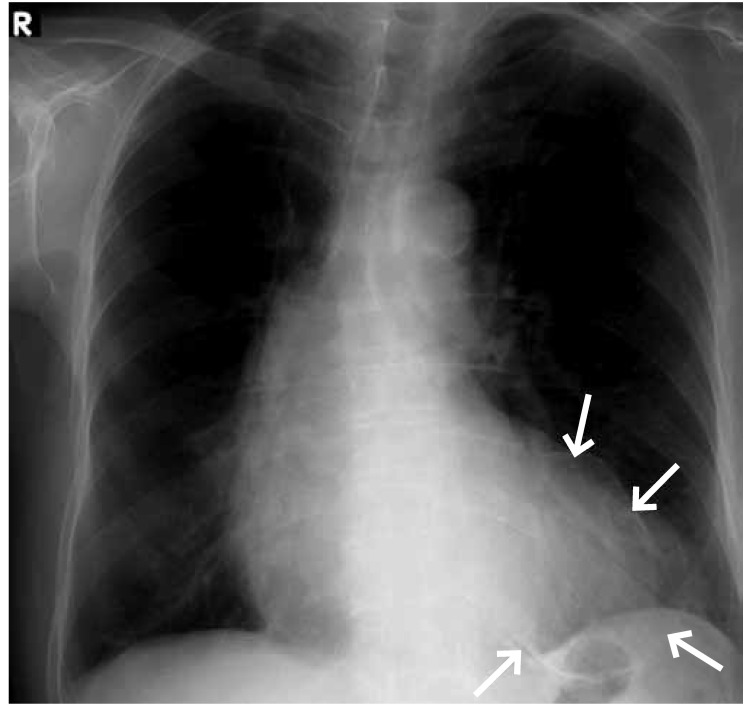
Thoracic X-ray showing an enlarged heart, and an oval-shaped calcified structure (arrows) related to a calcified antero-apical left ventricular aneurysm.
